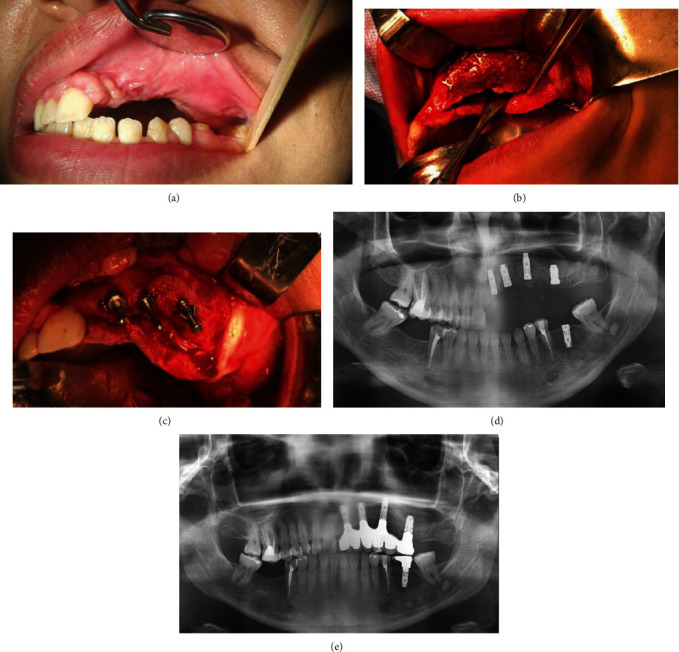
(a) Six months postoperation. (b) Exposing and removing the mesh. (c) Implant placement. (d) Radiograph of bone graft at 8-month follow-up. Note: the significant increase in bone augmentation of the maxilla and implant rehabilitation. (e) Complete regeneration in 3 years following reconstruction of the maxillary defect.
